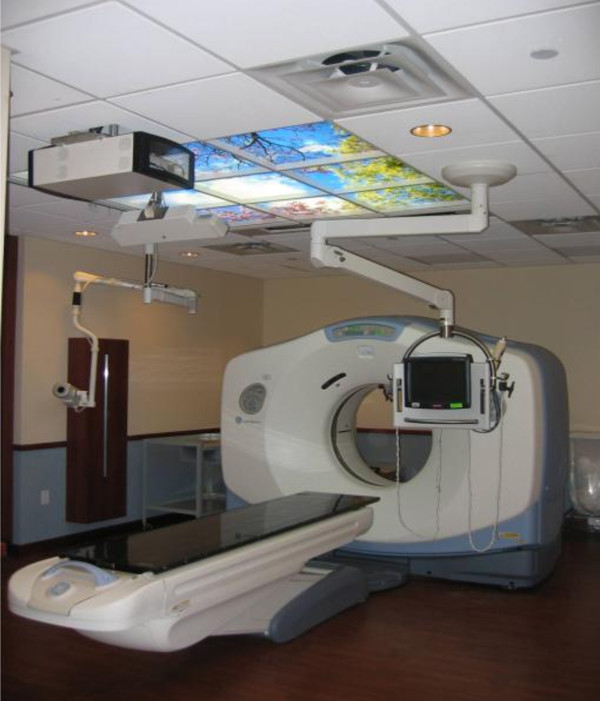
Clarity system: (a) US-Sim in CT room, and (b) US-Guide in treatment room.
